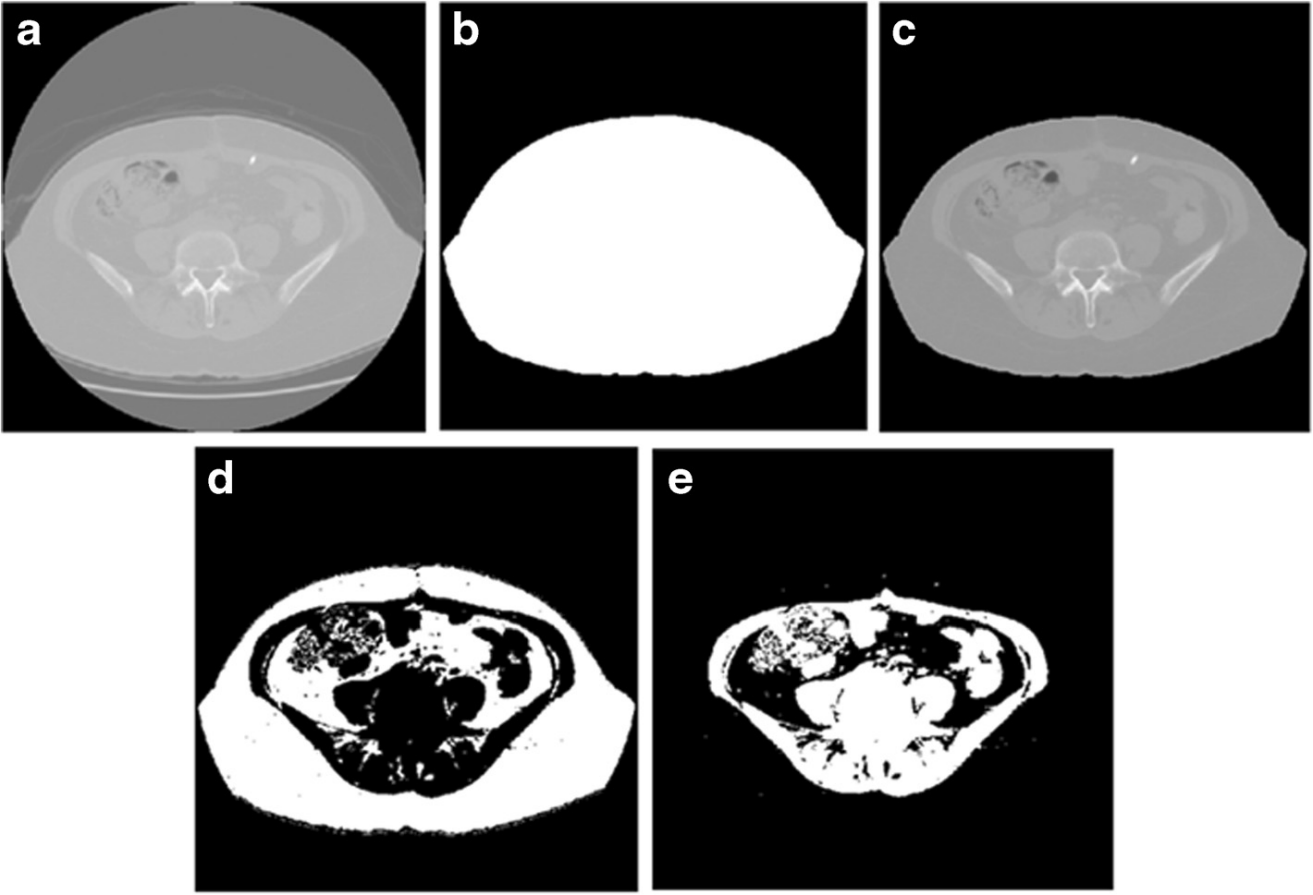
An example of applying our CAD scheme to segment a CT image slice. a An original CT image, b a CAD-generated body trunk mask, c segmented body region, d a CAD-generated fat region mask, and e a CAD-generated non-fat region mask
